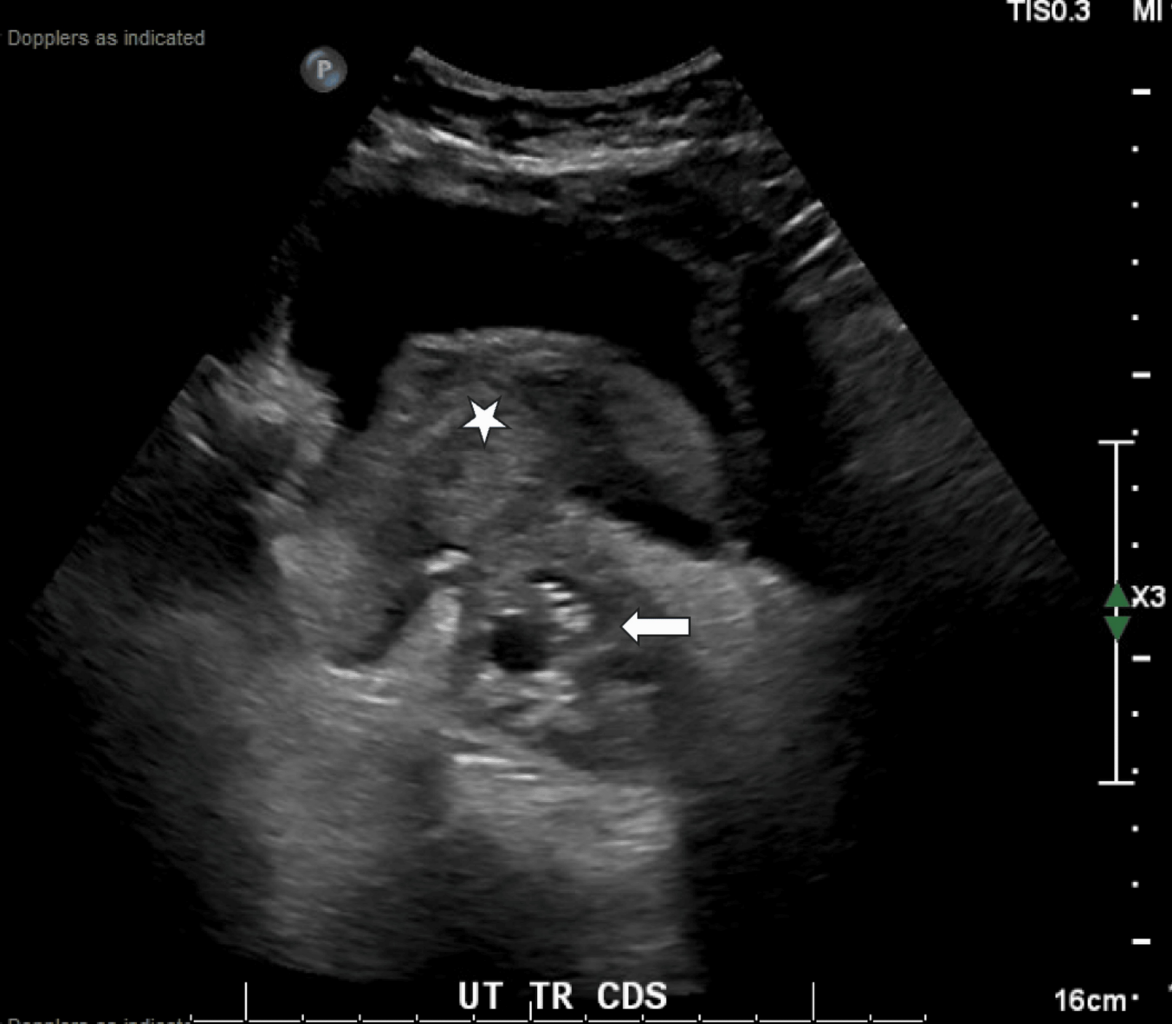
Large cervical mass with protrusion (white arrow) and clot burden in the bladder (white star) on a transvaginal ultrasound in the transverse plane
